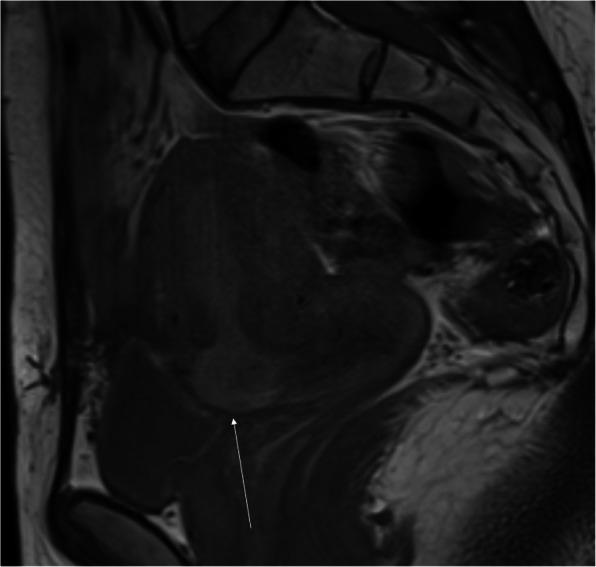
Pelvic MRI, SE/T1WI in the sagittal plane. Hyperintense blood reservoir in the C-section scar defect with intact serosa (arrow), which is isointense to the myometrium
